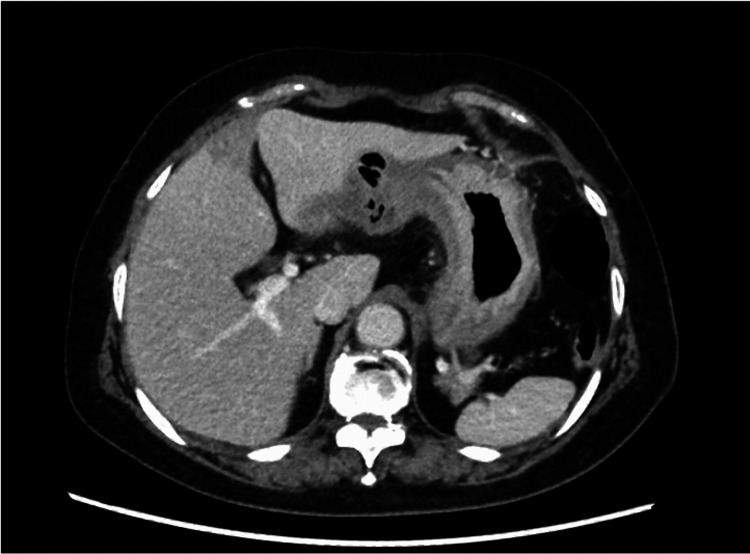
CT scan of the abdomen showed the stomach wall was thickened.
